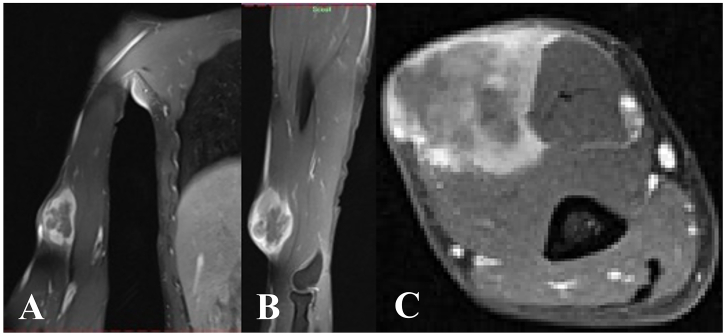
MRI of the tumor. A) Coronal view, B) Sagittal view, C) Transversal view.
